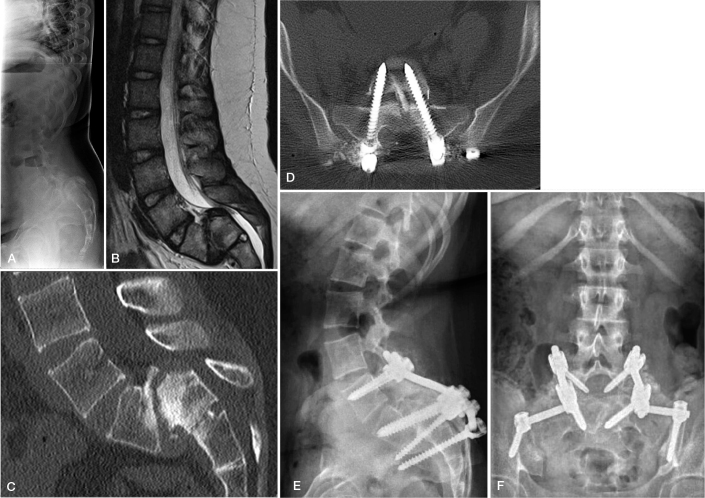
Spondyloptosis in a 12-year-old girl with bilateral L5 radicular symptoms. (A) Standing radiograph shows pelvic retroversion. (B) Sagittal T2 weighted MR image shows spondyloptosis with narrowing central spinal canal. (C) Sagittal reformatting of lumbar CT shows remodeling of the S1 endplate with residual apophyseal growth plate. (D) Axial CT image with trans-sacral screw fixation with a non-vascular fibular strut. (E) Standing lateral and (F) posteroanterior radiograph at 2-year follow-up shows bridging bone formation posterolaterally and reduced pelvic retroversion.
